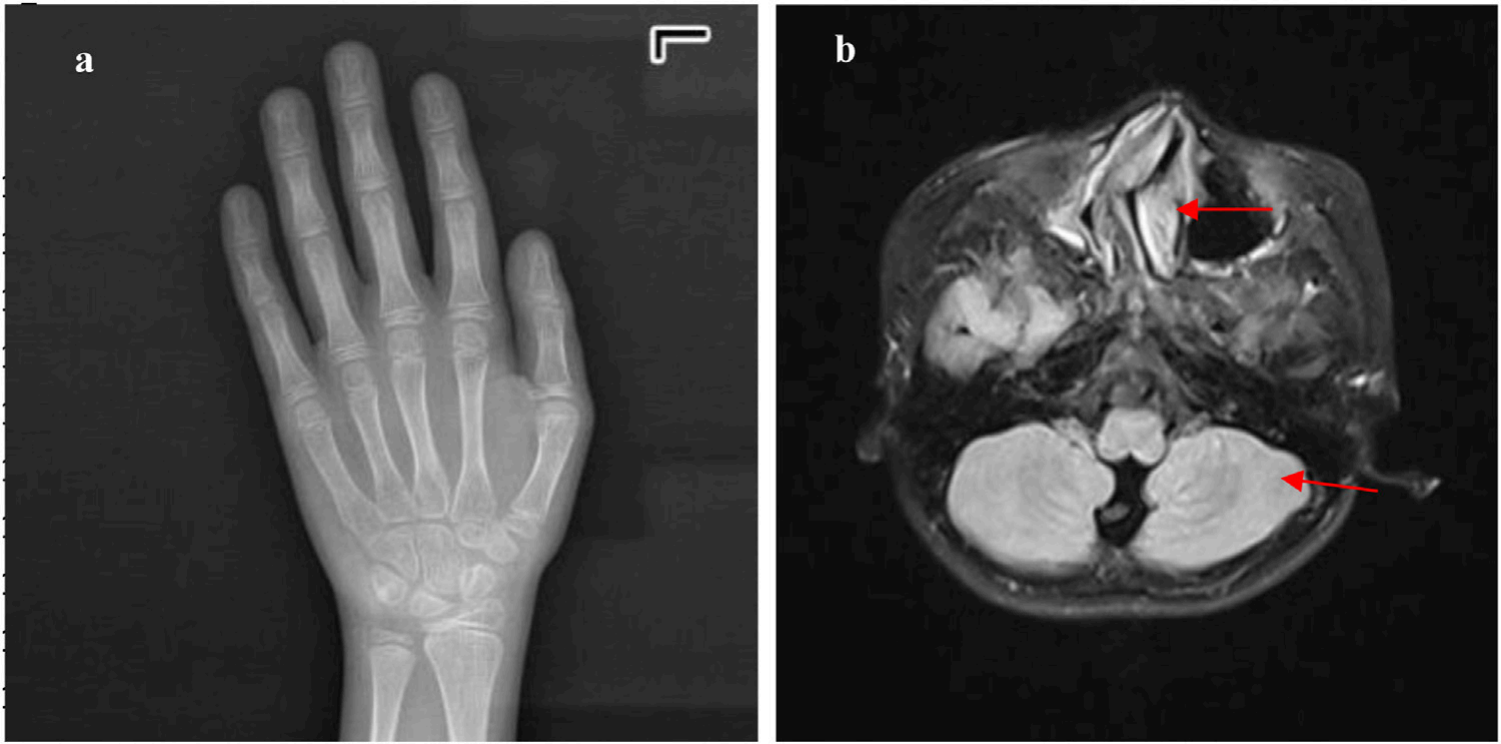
The metacarpal and phalangeal bones and cranial MRI of Case 1: (a) shows a bone age of 11 years, with an actual age of 10 years and 9 months, indicating the bone age is neither advanced nor delayed. (b) shows that the cranial MRI indicates slightly widened cerebellar sulci on the left side (red arrow), a markedly rightward deviation of the nasal septum in the middle (red arrow), and hypertrophy of the bilateral inferior nasal turbinates.
